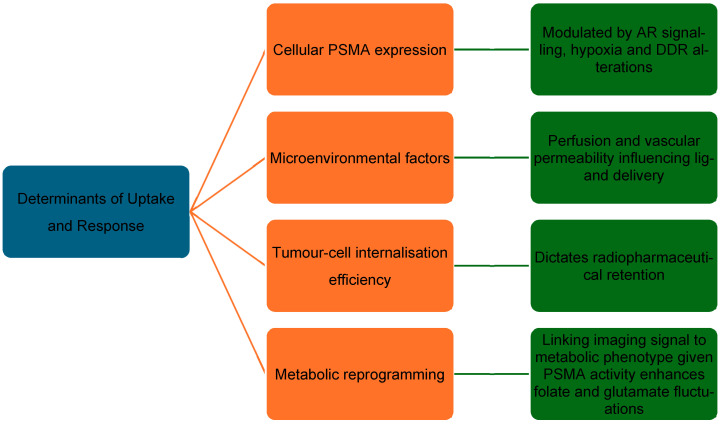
Determinants of PSMA uptake and response. DDR: DNA damage response.
